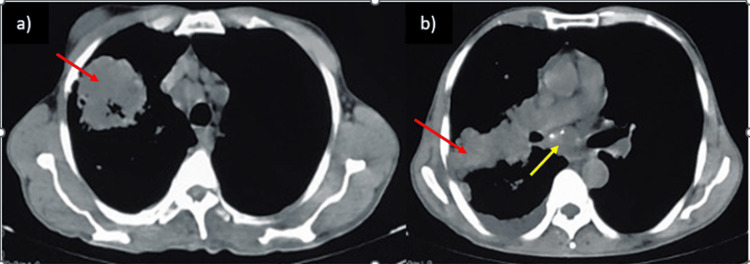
Mediastinal window of high-resolution computed tomography of thorax. (a) Ill-defined necrotic soft tissue mass lesion (red arrow) in apical segment of right upper lobe of 5.9 cm × 5.6 cm × 5.6 cm; (b) medial extension of mass into right hilum and lateral extension to the chest wall (red arrow) is seen along with an enlarged subcarinal mediastinal lymph node (yellow arrow).
